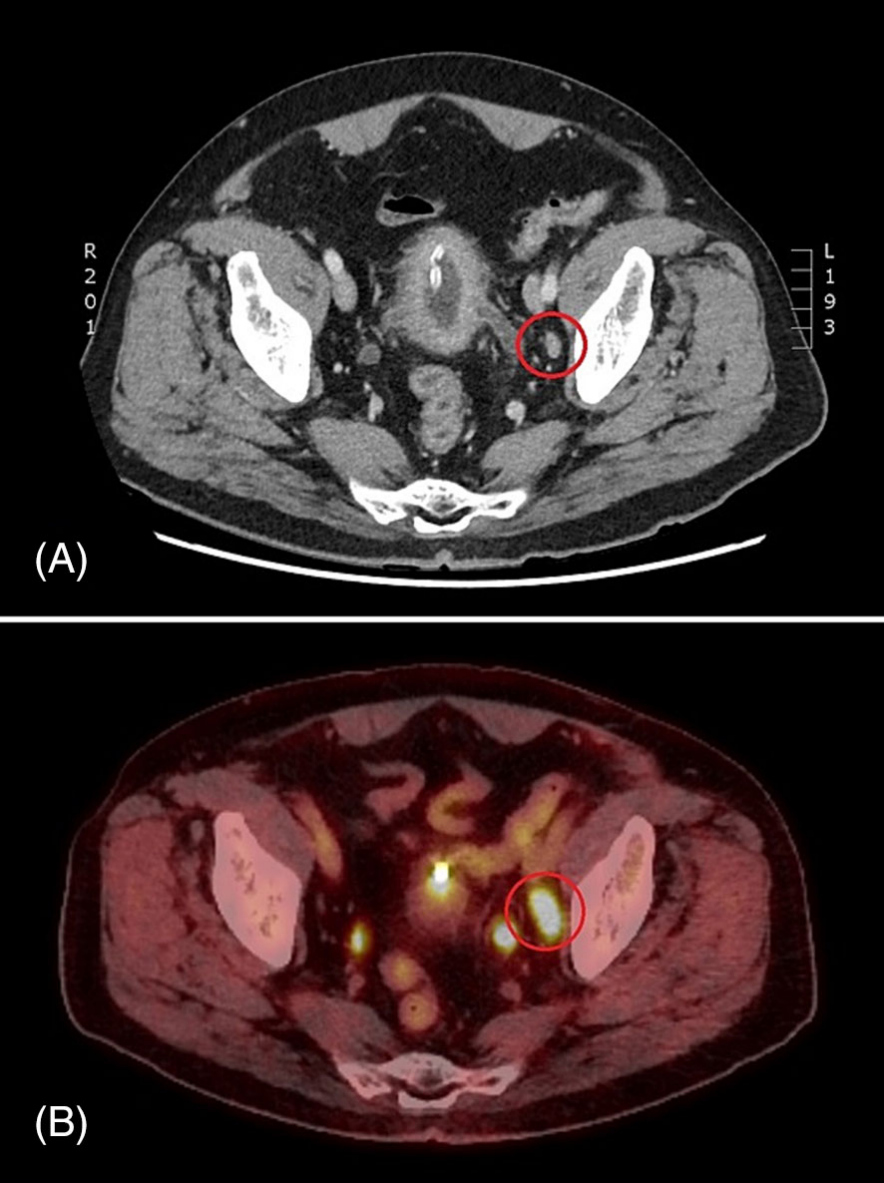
CT and FDG PET of the same patient (<8 weeks apart) showing (A) a negative CT as per RECIST criteria [small left external iliac lymph node in CT (8 mm)] and (B) a positive FDG PET showing same LN with SUV max 9.9 (SUVmax >4).
